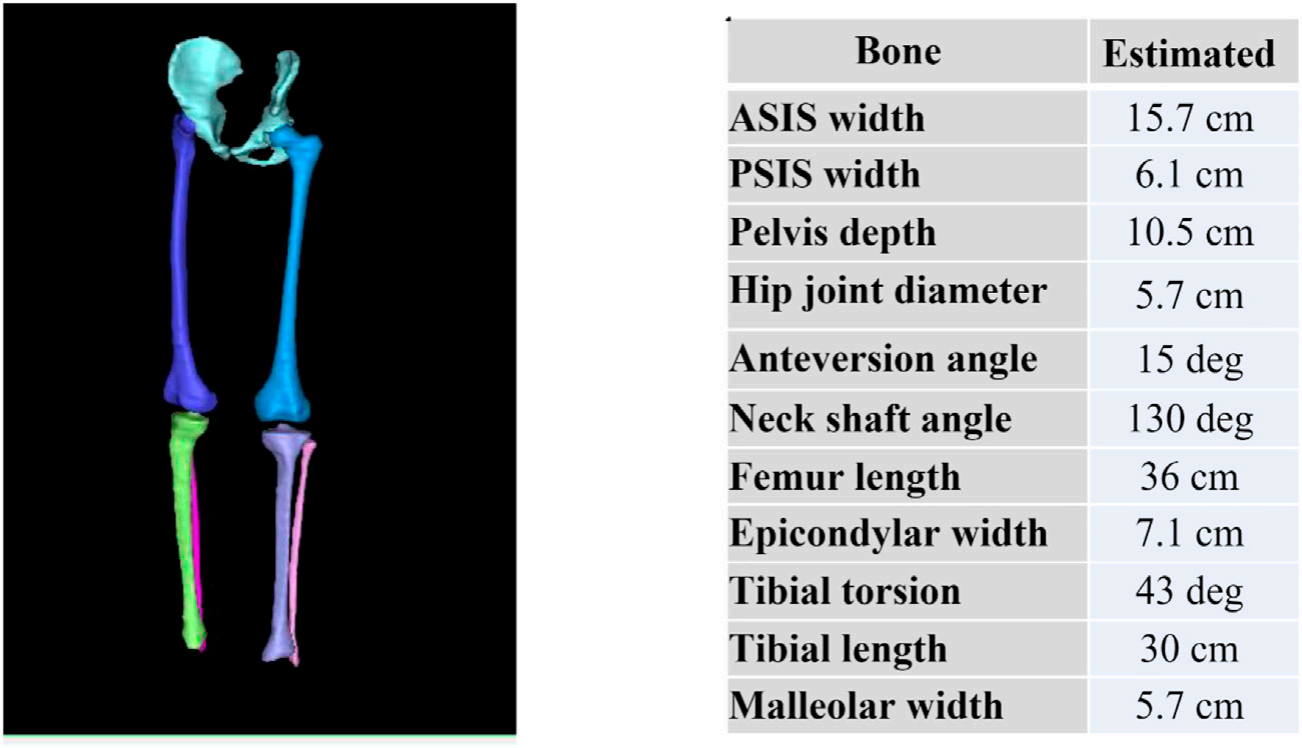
Reconstructed pelvis, femurs, tibias and fibulas from MRI and subsequently fitted with template meshes (left) to automatically compute bone torsional and linear measurements (right).
